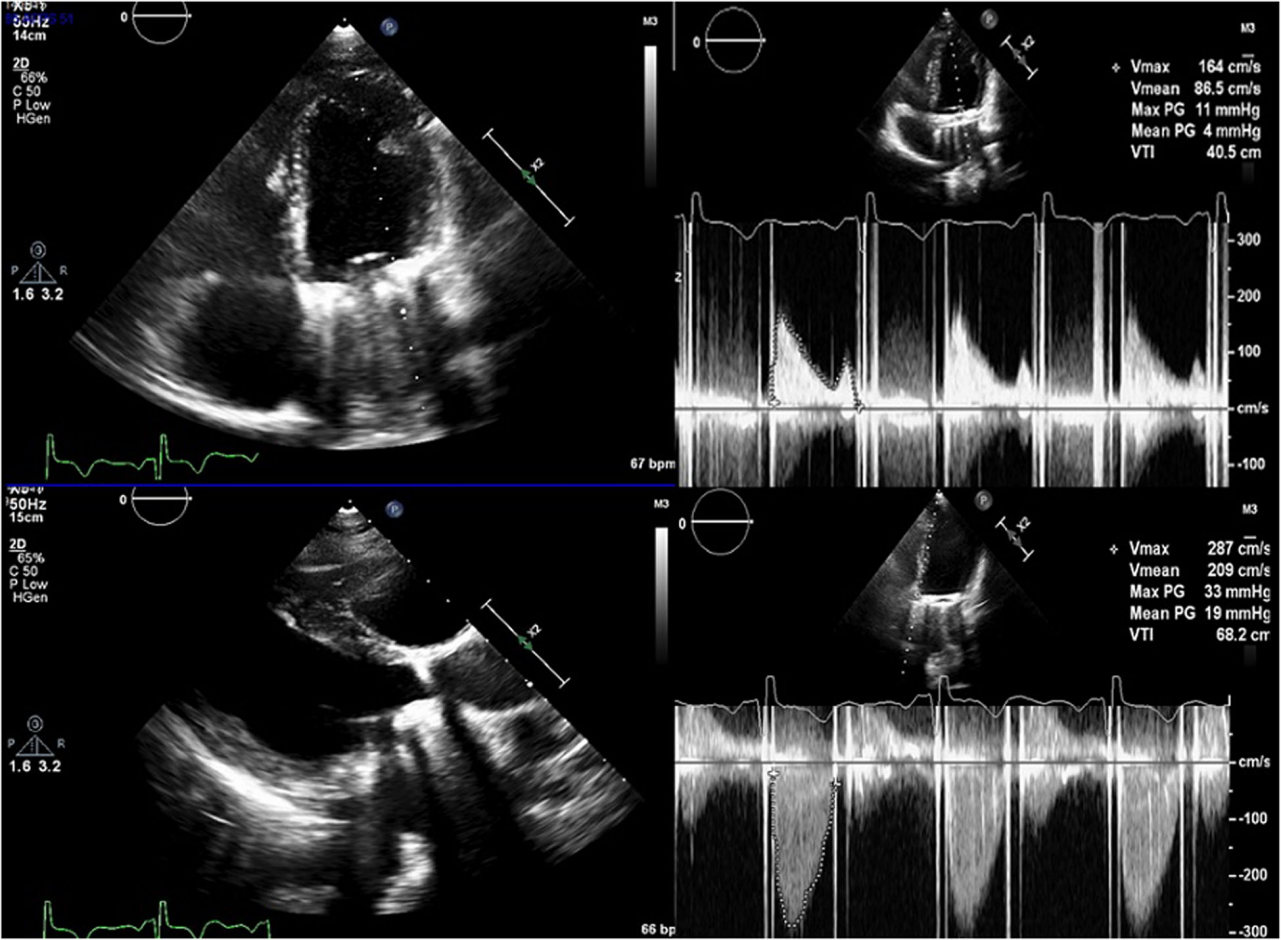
Parasternal Long Axis Echocardiographic view shows Mechanical Aortic and Mitral Valve with no identifiable thrombus formation. Parameters of Mechanical Aortic and Mitral Valve as seen apical 4 and 5 chamber echocardiographic view
